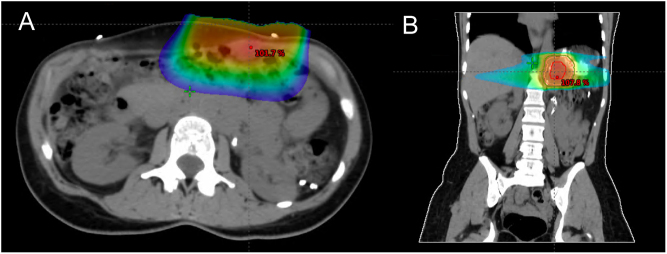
Axial (A) and coronal (B) CT imaging of radiation therapy planning targeting the anterior abdominal wall recurrence (A) and the retroperitoneal mass at the initial tumor bed (B).
